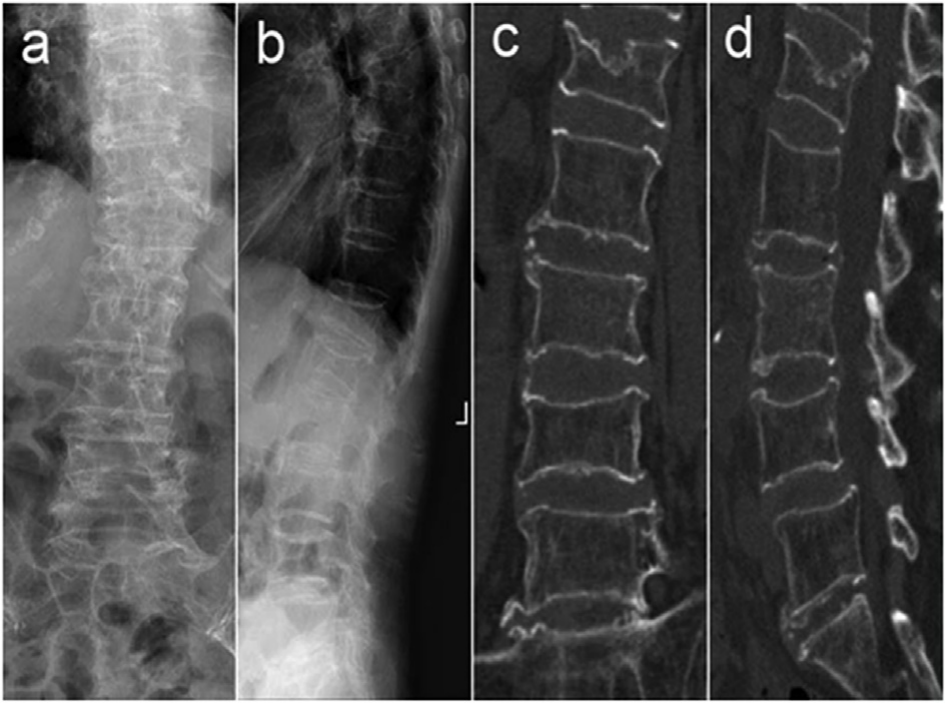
Preoperative X-ray (a, b), and crown-lost CT reconstruction (c, d), suggest T7, T12, L1 multiple vertebral compression fractures.
